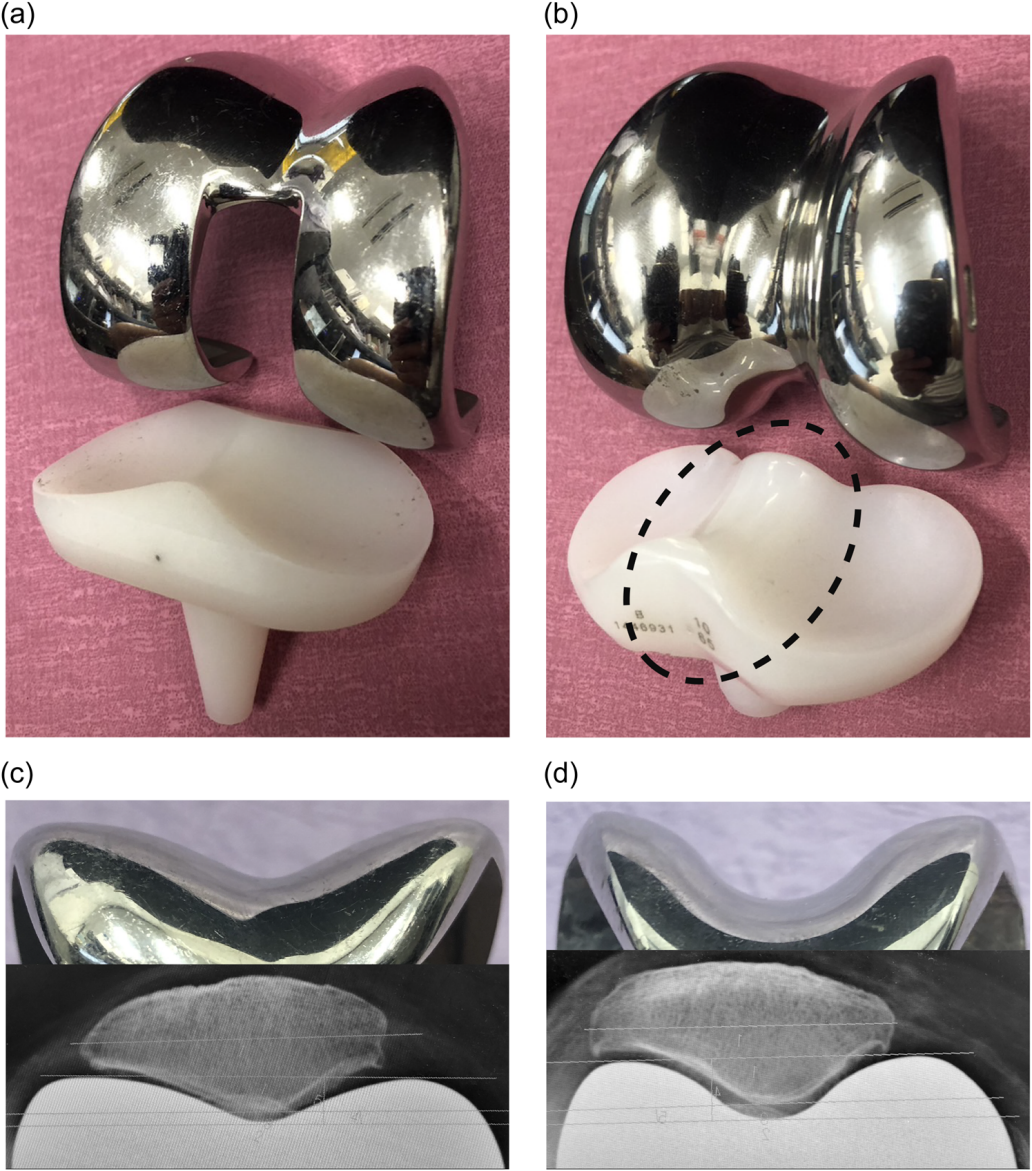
Comparison of the appearance of LCS‐RP with that of ROCC, shown by actual implants and axial radiographs. (a) Appearance of LCS‐RP: femoral component with a deep anatomical trochlear groove and an RP insert with a high anterior lip. (b) Appearance of ROCC: RP inserts with a hyperbolic paraboloid (saddle‐shaped) prominence (dotted ellipse) and femoral component with a deep trochlea that receives the saddle‐shaped prominence. (c) Femoral trochlea of LCS‐RP: the native patella fits into the anatomically shaped femoral trochlea of LCS‐RP. (d) Femoral trochlea of ROCC: the native patella fits more deeply into the deeper femoral trochlea of ROCC than that of LCS‐RP. LCS, low contact stress; ROCC, rotating concave–convex; RP, rotating platform.
